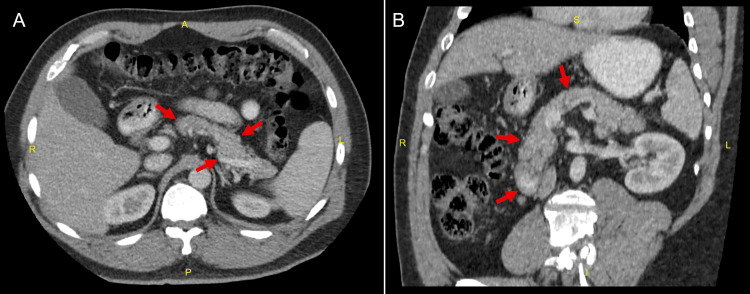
Abdominal CT findings. (A) Axial and (B) coronal images demonstrate a pancreas of normal size and morphology without an identifiable focal pancreatic lesion (red arrows). CT, computed tomography. Orientation markers: A, anterior; P, posterior; R, right; L, left; S, superior; I, inferior.
